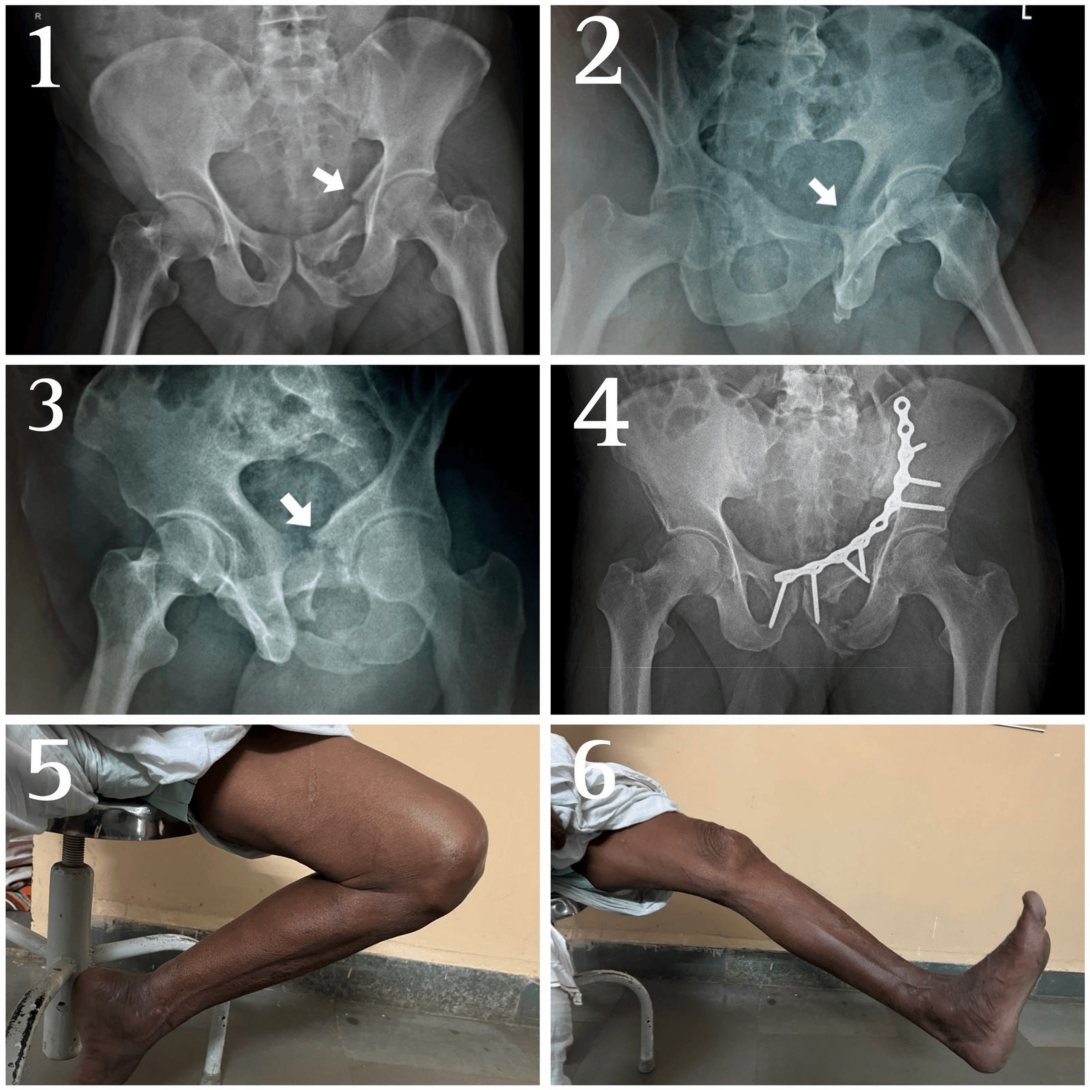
Pre- and postoperative X-rays of patient with anterior column fracture using modified Stoppa approach Pre-operative radiographs—first: Anteroposterior (AP), second: Iliac oblique (IO), and third: Obturator oblique (OO) views with arrows showing the fracture. Fourth: Immediate postoperative radiographs; fifth and sixth: Clinical photographs of patients at six-month follow-up
